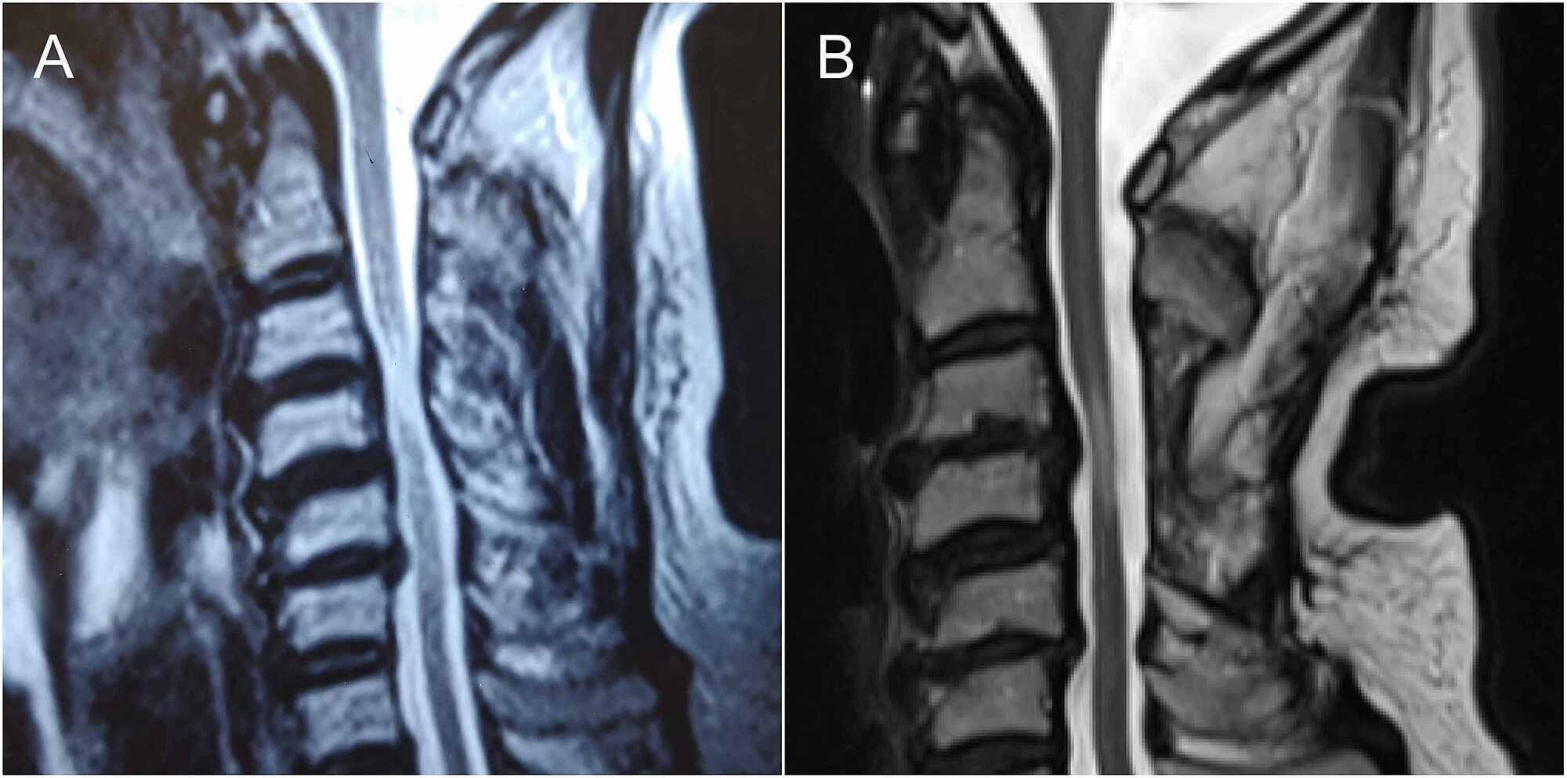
Pre- and postoperative cervical MRI Sagittal MRI T2 weighted. (A) Preoperative image displaying cervical spondylosis characterized by loss of lordosis, disc bulging, and cervical stenosis predominately at C3-4. A hyperintense signal is observed in the spinal cord at C3-4. (B) Postoperative image showing adequate decompression of the cord at C3-4, note the persistence of the hyperintense signal in the same area of the cord.
